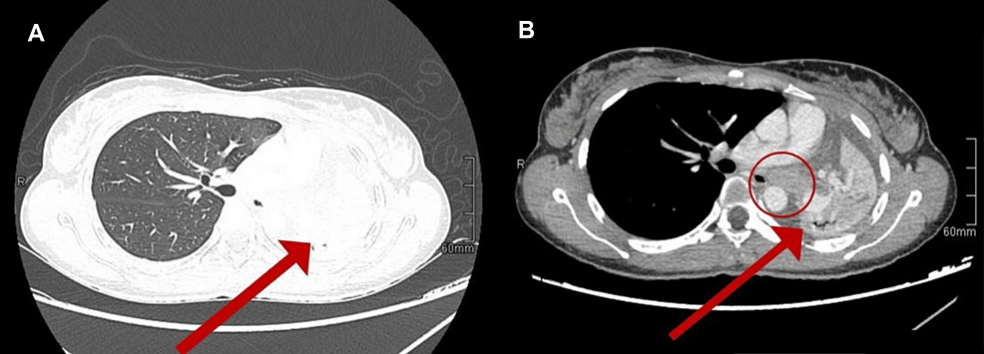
Axial chest CT without contrast (A) and with contrast (B) showing near total lung collapse (red arrow) showing lesions 30x19 mm (red circle) in the left main bronchus with bronchial obstruction
